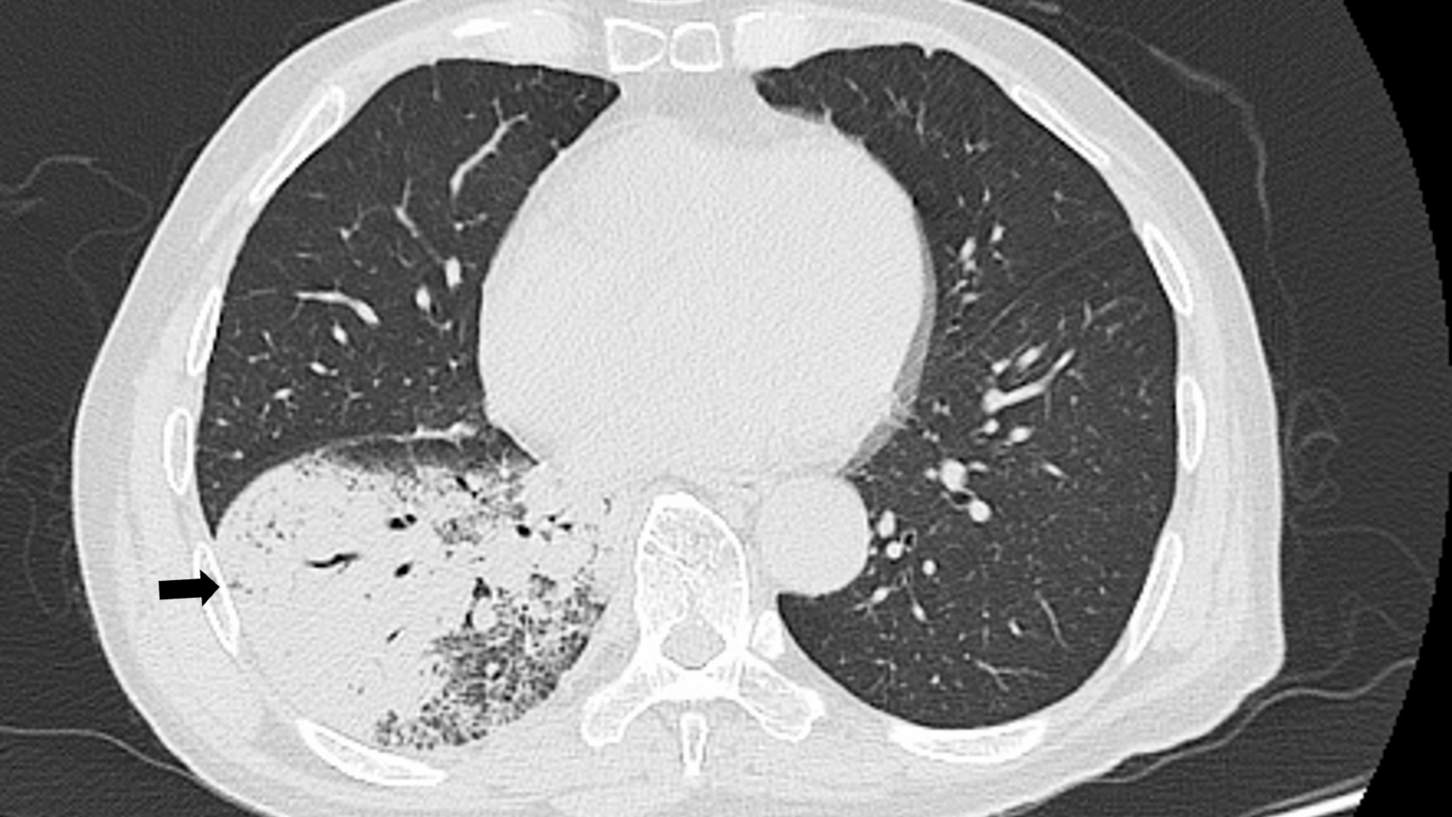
Chest CT on first admission showing consolidation with ground-glass opacities in the right lower lobe, consistent with Legionella pneumonia (arrow).
